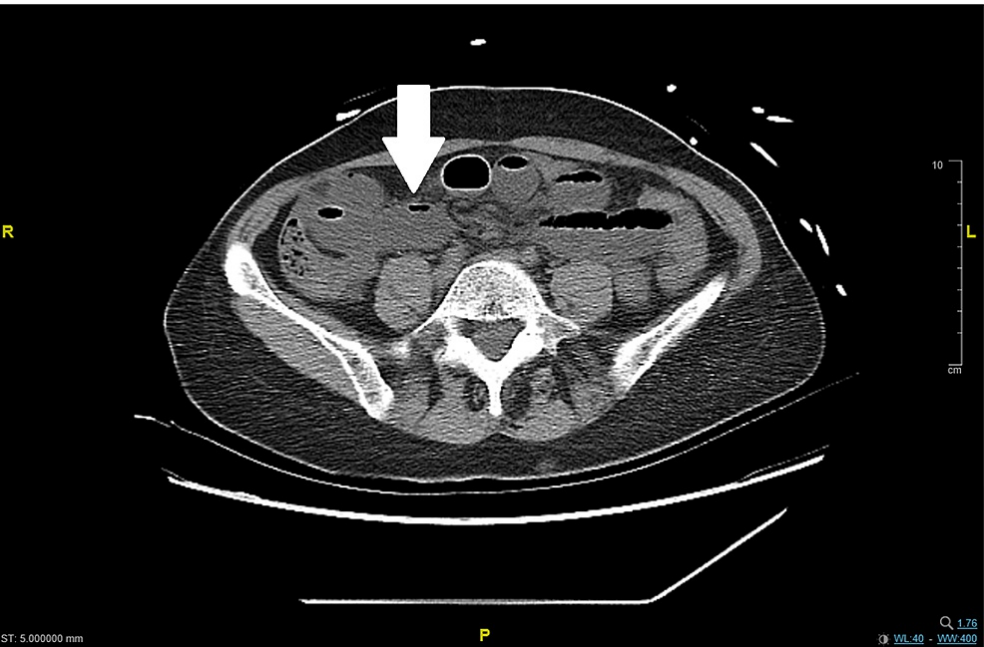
Computed tomography (CT) scan of the abdomen showing dilated intestinal loops (white arrow)
